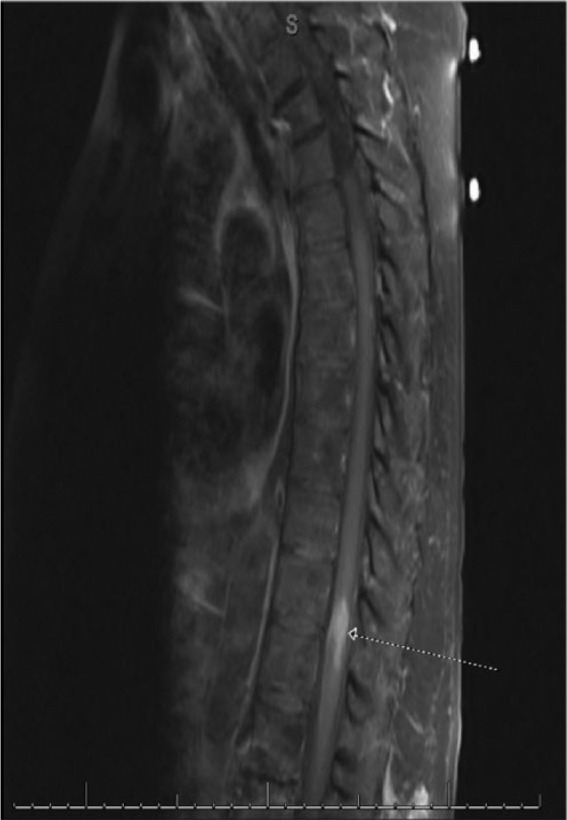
Sagittal spinal MRI shows an enhancing intramedullary lesion of the thoracic spinal cord at T11-T12.
